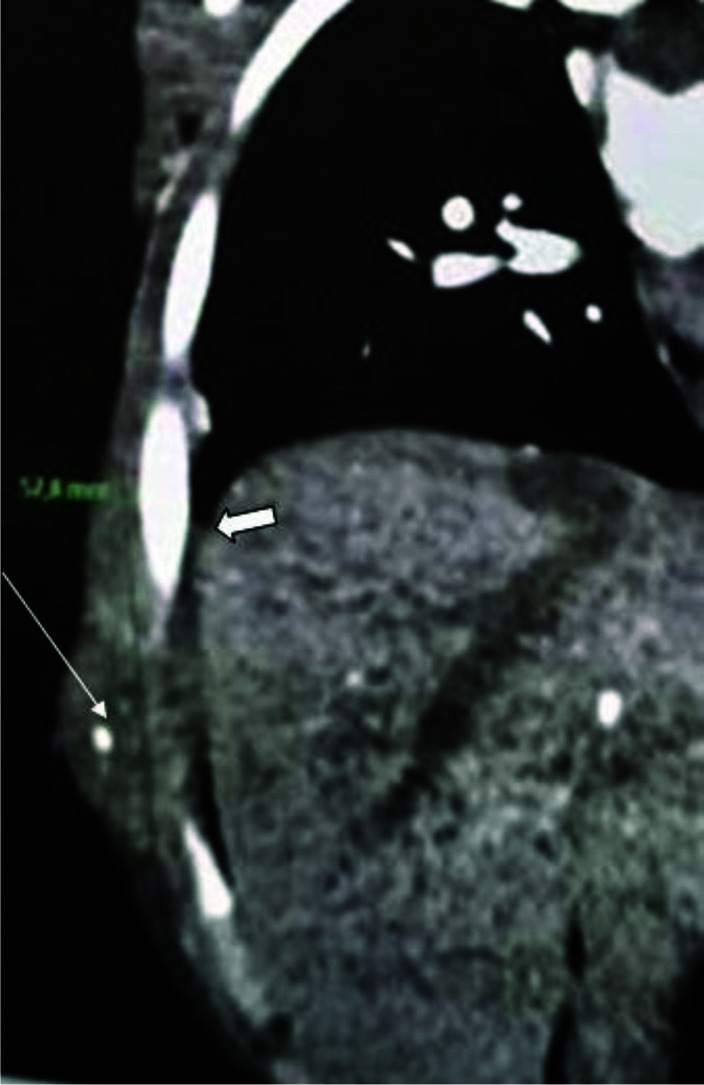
Coronal thoraco-abdominal computed tomography with a mediastinal window showing a 5×6 cm empyema eroding into the right anterior chest wall (arrowhead) associated with pleural densification (large arrow) confirming the diagnosis of empyema necessitans.
